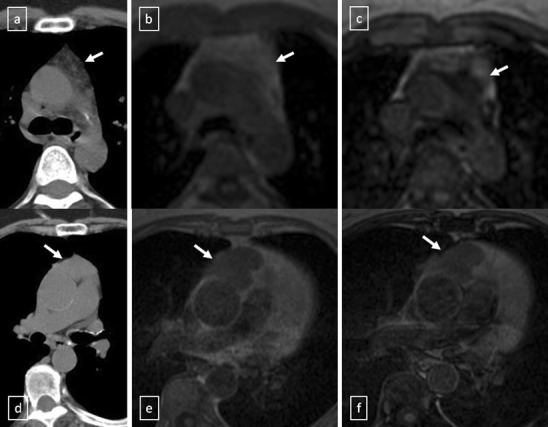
a–c* Thymic hyperplasia in a 43-year-old woman (*arrows*). a Non-contrast-enhanced CT scan reveals a lobulated lesion with smooth margins in anterior mediastinum. b Lesion appears slightly hyperintense on in-phase gradient-echo T1-weighted MR image. c Opposed-phase gradient-echo T1-weighted MR image shows decreased signal intensity within the lesion, confirming presence of fat. *d–f* Stage II thymoma (WHO type B2) in a 62-year-old woman (*arrows*). d Non-contrast-enhanced CT scan shows an anterior mediastinal soft tissue mass. e The lesion shows an intermediate signal intensity on in-phase gradient-echo T1-weighted MR image. f There is no substantial decrease in signal intensity relative to in-phase MR image on opposed-phase sequence
